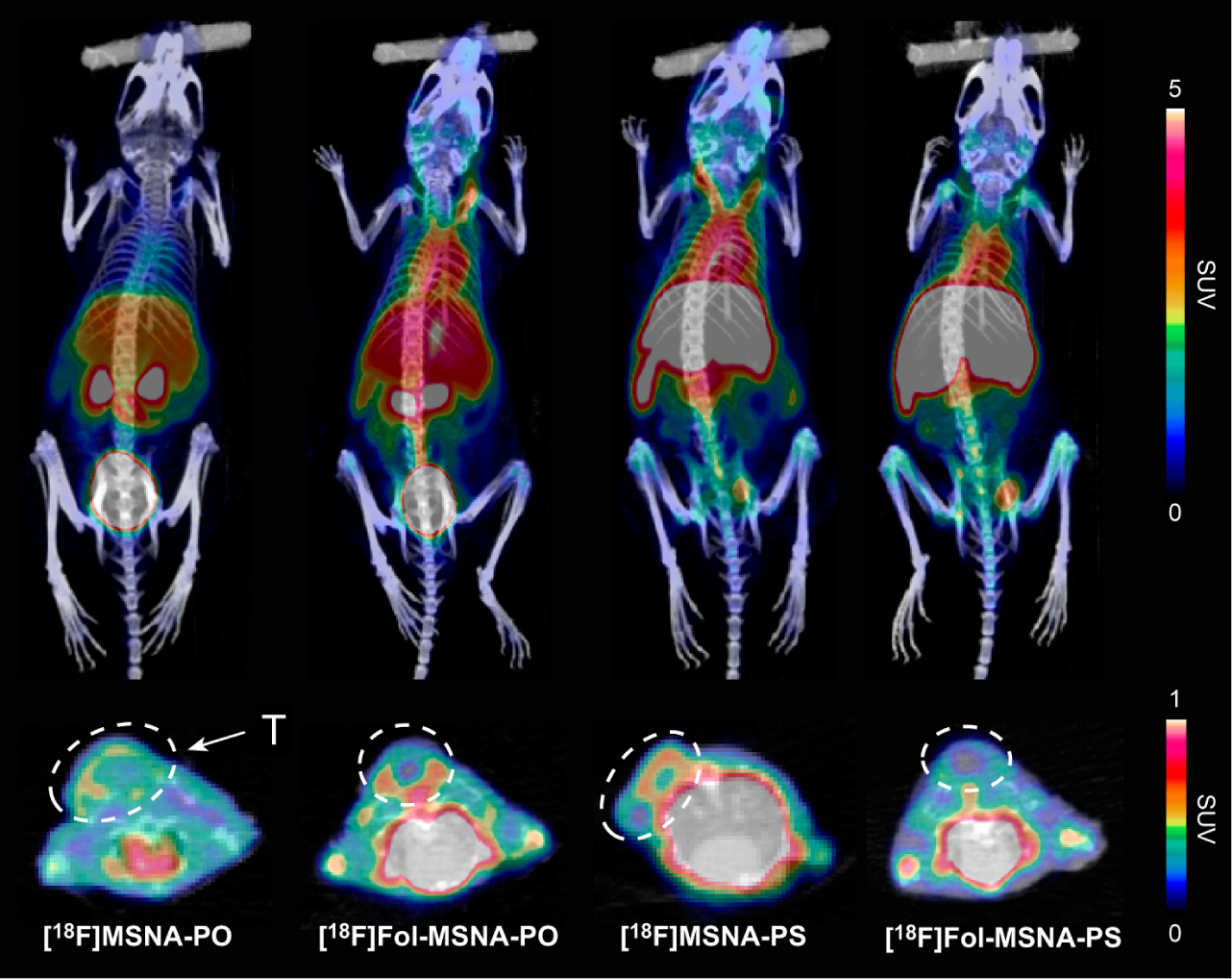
PET/CT images (maximum intensity projections in the coronal
